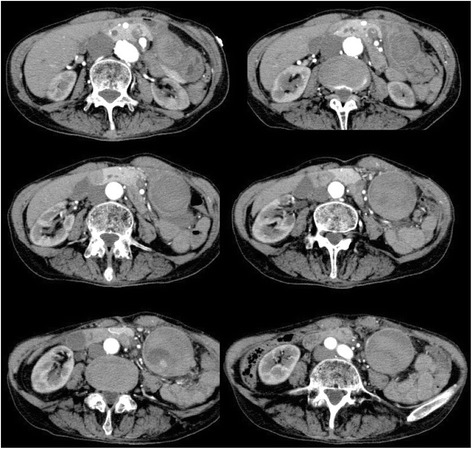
Contrast-enhanced computed tomography showed a large mass of 5 × 5 × 8 cm in the left middle abdominal quadrant. The mass was found to be attached to the gastric wall
